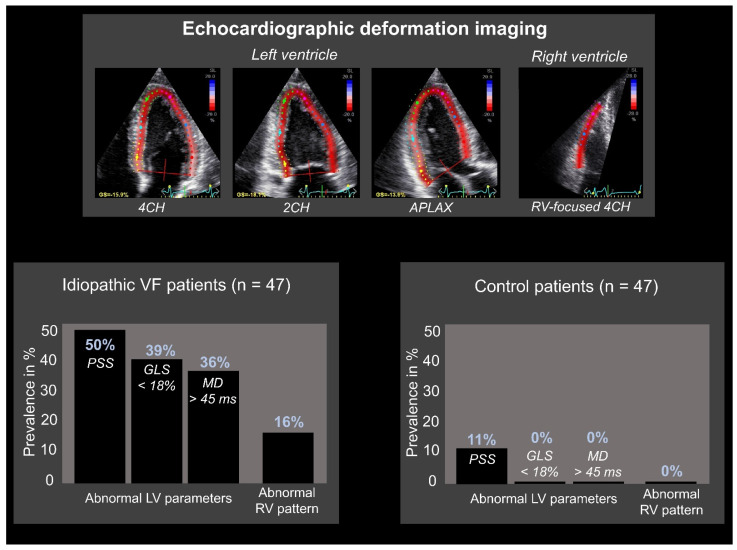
Echocardiographic deformation imaging in patients with idiopathic ventricular fibrillation. Abnormal results were primarily found in idiopathic ventricular fibrillation (IVF) patients compared to healthy controls. Global deformation imaging includes left ventricular (LV) global longitudinal strain (GLS): defined as “the average global peak strain from three apical views” and LV mechanical dispersion (MD): defined as “the standard deviation of time to peak longitudinal strain from the 18 LV segmental deformation curves”. LV GLS < 18% was considered abnormal, LV MD > 45 ms was considered abnormal. Regional deformation imaging includes postsystolic shortening (PSS): defined as “longitudinal myocardial shortening after aortic valve closure”. PSS ≥ 10% was considered abnormal. 2CH: 2-chamber; 4CH: 4-chamber; APLAX: apical long axis; RV: right ventricular. Adapted with permission from Groeneveld et al. [45].
